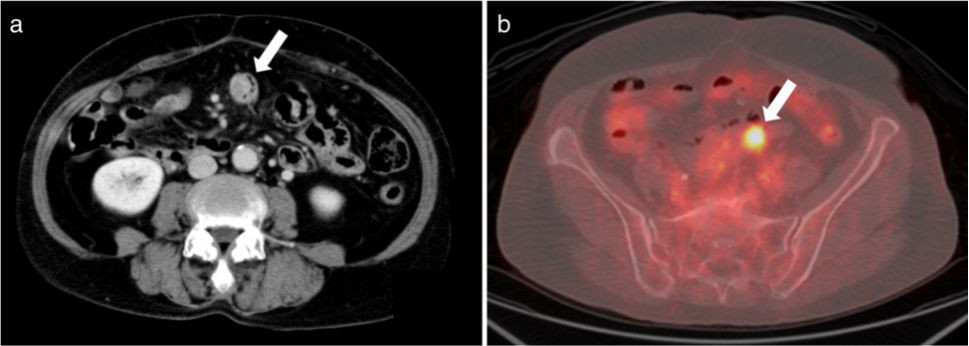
Computed tomography showed a mass measuring 20 × 20 mm in the small intestine (a) (*arrow*), and ^18^F-fluorodeoxyglucose (^18^FDG)-positron emission tomography/computed tomography showed an abnormal uptake of ^18^FDG in the lesion (b) (*arrow*)
